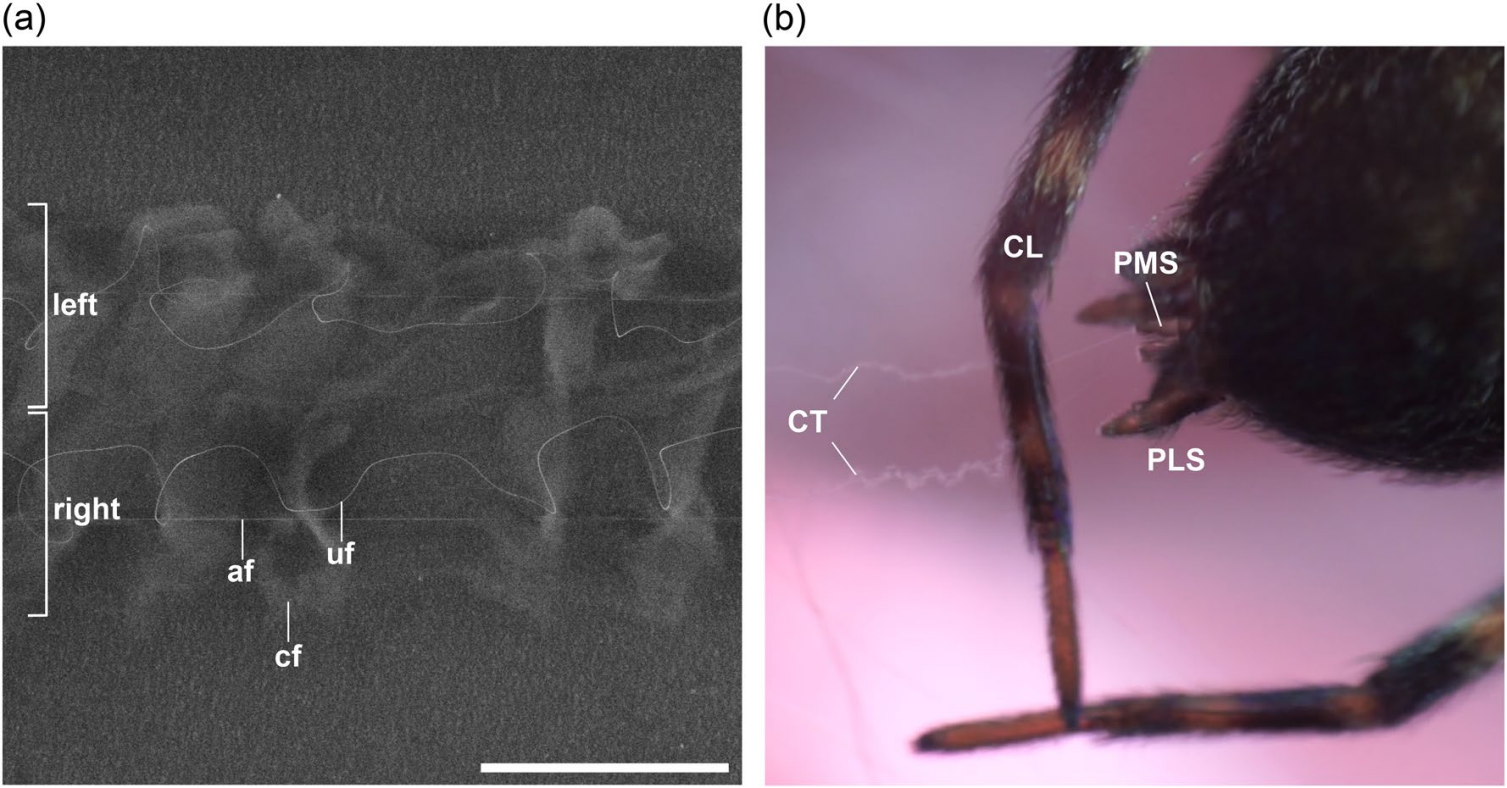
Capture thread of *B.* *longinqua* and its formation. The thread consisted of two strands, which could be either a closely parallel or b separate. a Each strand (left and right half) contained one axial fibre (af), one undulating fibre (uf) and many cribellate fibres (cf) formed into a mat; Scale bar 200 µm. b The halves of the cribellate thread (CT) were produced separately right from the beginning. The emergence of individual fibres could be seen at the posterior lateral (PLS) and posterior median (PMS) spinnerets. The final thread structure became visible after processing by the combing leg (CL). af: axial fibre, cf: cribellate fibres, CL: combing leg, CT: cribellate thread, PLS: posterior lateral spinneret, PMS: posterior median spinneret, uf: undulating fibre
